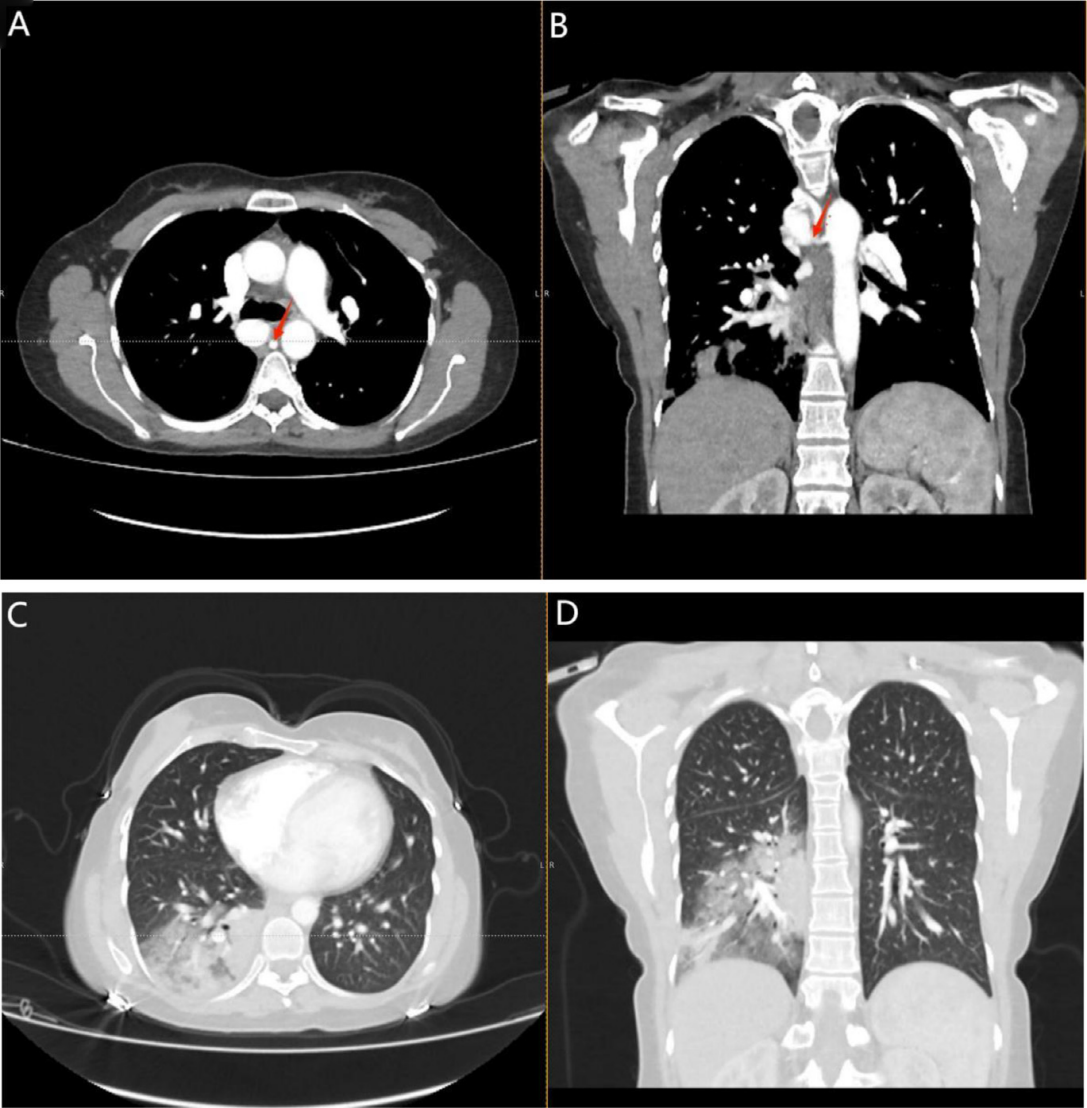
(A) Axial contrast-enhanced CT angiography of the chest showing a right bronchial artery aneurysmal dilatation measuring approximately 2.3 × 3.6 cm (red arrow). (B) Coronal contrast-enhanced CT angiography of the chest showing a right bronchial artery aneurysmal dilatation measuring approximately 2.3 × 3.6 cm (red arrow). (C) Axial noncontrast lung window CT of the chest showing Right lower zone consolidation with air bronchogram in keeping with alveolar airspace disease suggestive of pulmonary hemorrhage or infection. (D) Coronal noncontrast lung window CT of the chest showing Right lower zone consolidation with air bronchogram in keeping with alveolar airspace disease suggestive of pulmonary hemorrhage or infection.
